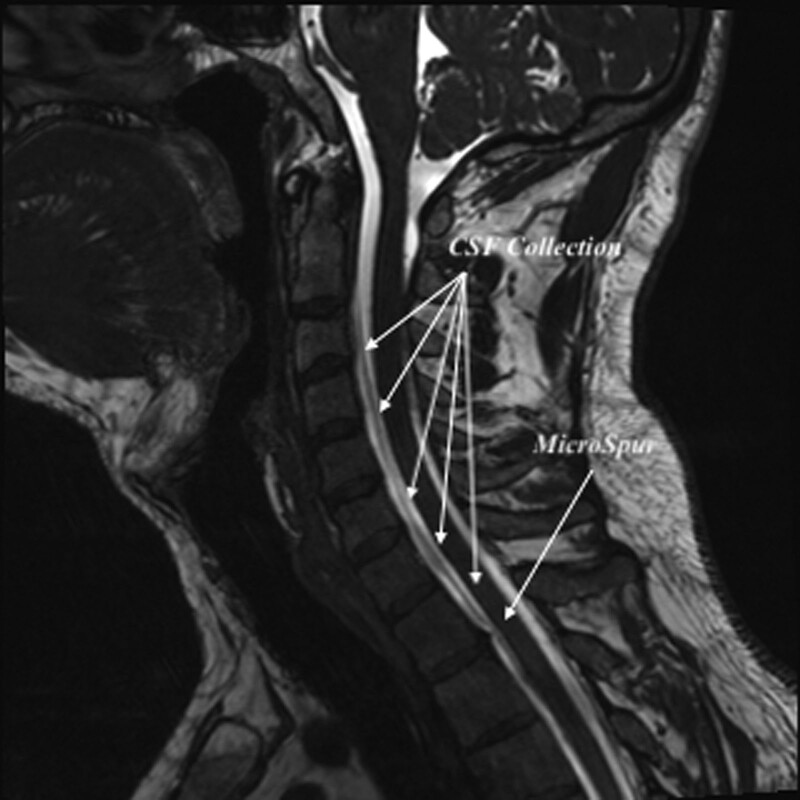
Full-spine magnetic resonance imaging.
